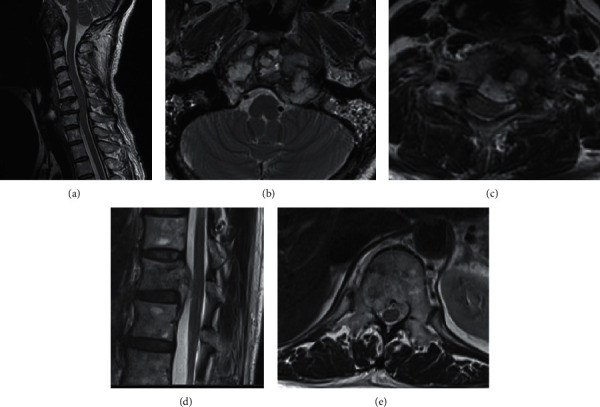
MRI at first presentation. (a) Sagittal image of tumors at skull base and C6, axial image of tumor at (b) skull base and (c) C6, and (d) sagittal and (e) axial images of the tumor at T12.
